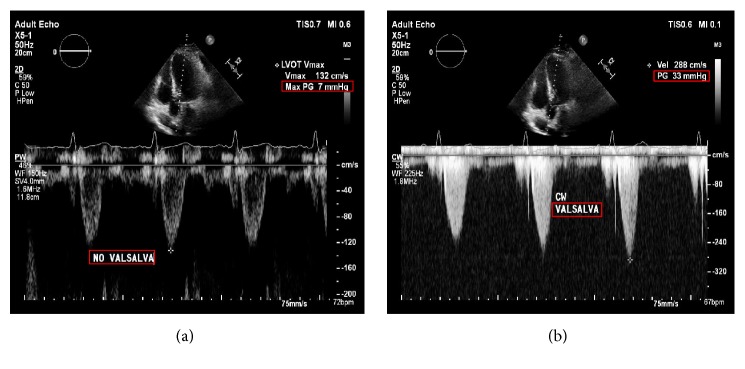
Postoperative Transthoracic Echocardiogram demonstrating resting left ventricular outflow tract pressure gradient of 7 mmHg (a) and a pressure gradient of 33 mmHg on Valsalva (b).
